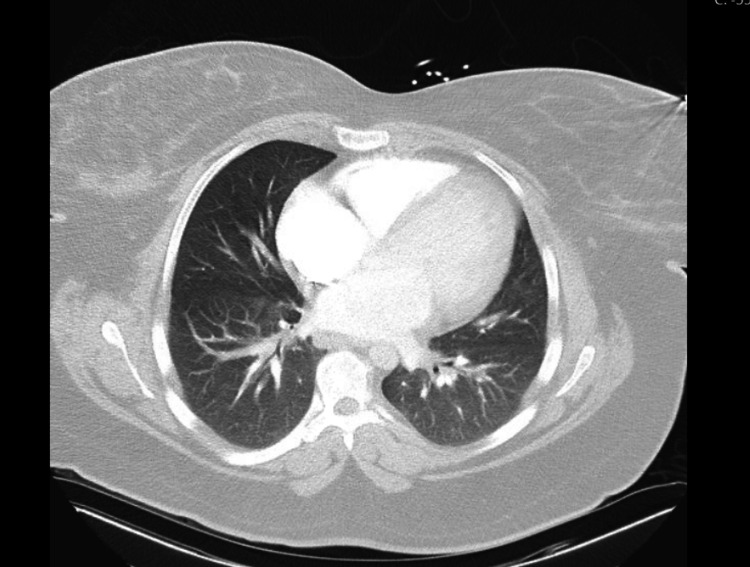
CT angiogram obtained on admission
